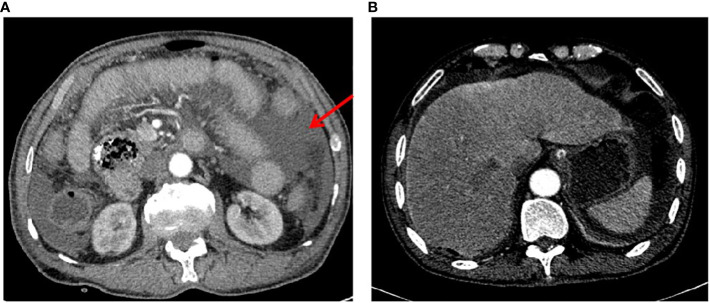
Preoperative enhanced CT scan images. (A) A typical image highlighting the presence of effusion in the abdominal cavity, as marked by the red arrow. (B) A representative image demonstrating the absence of metastatic lesions in the liver.
